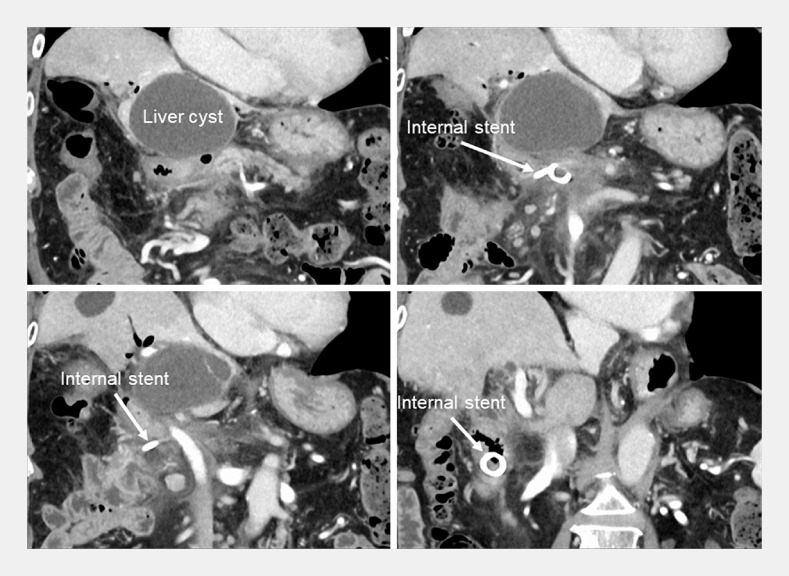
CT taken 2 months following drainage showing complete disappearance of the fluid collection.
